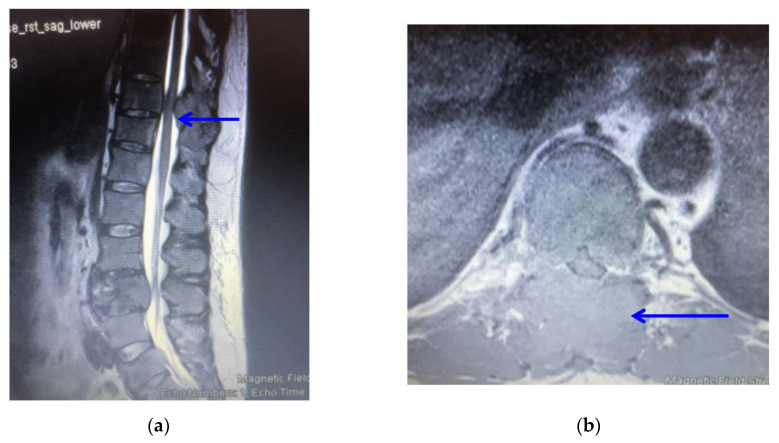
(a): T2-weighted sagittal sequence MRI scan showing an epidural soft tissue tumour compressing the spinal cord (blue arrow); (b): T2-weighted axial sequence MRI scan at T10 showing marked compression of the spinal cord from a soft tissue tumour (blue arrow).
